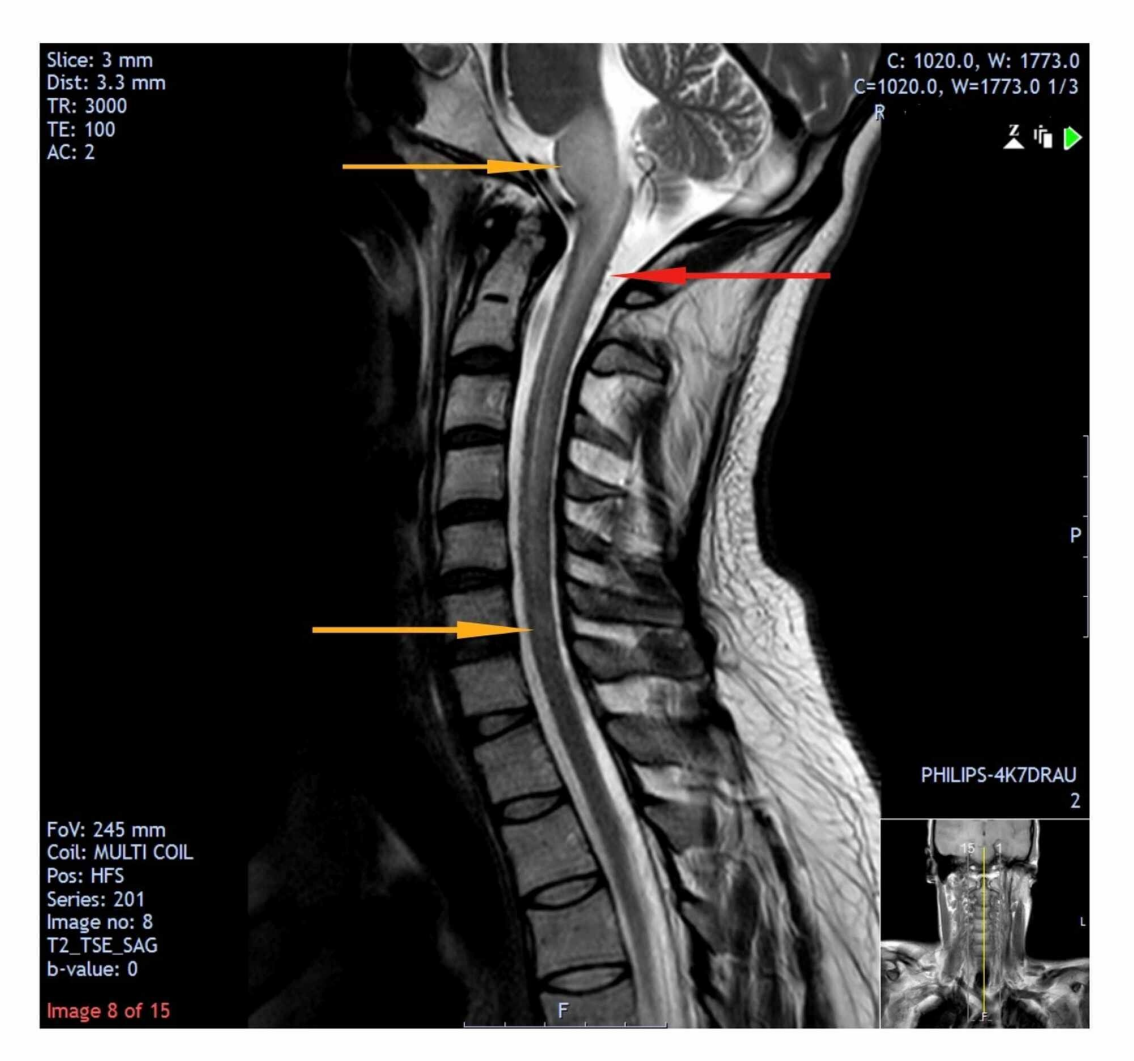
T2-weighted MRI scan showing the extent of spinal cord oedema (orange arrows) Signal change seen especially in the medulla and upper cervical cord (red arrow). This could be an indirect finding to suggest the presence of a dural arteriovenous fistula.
